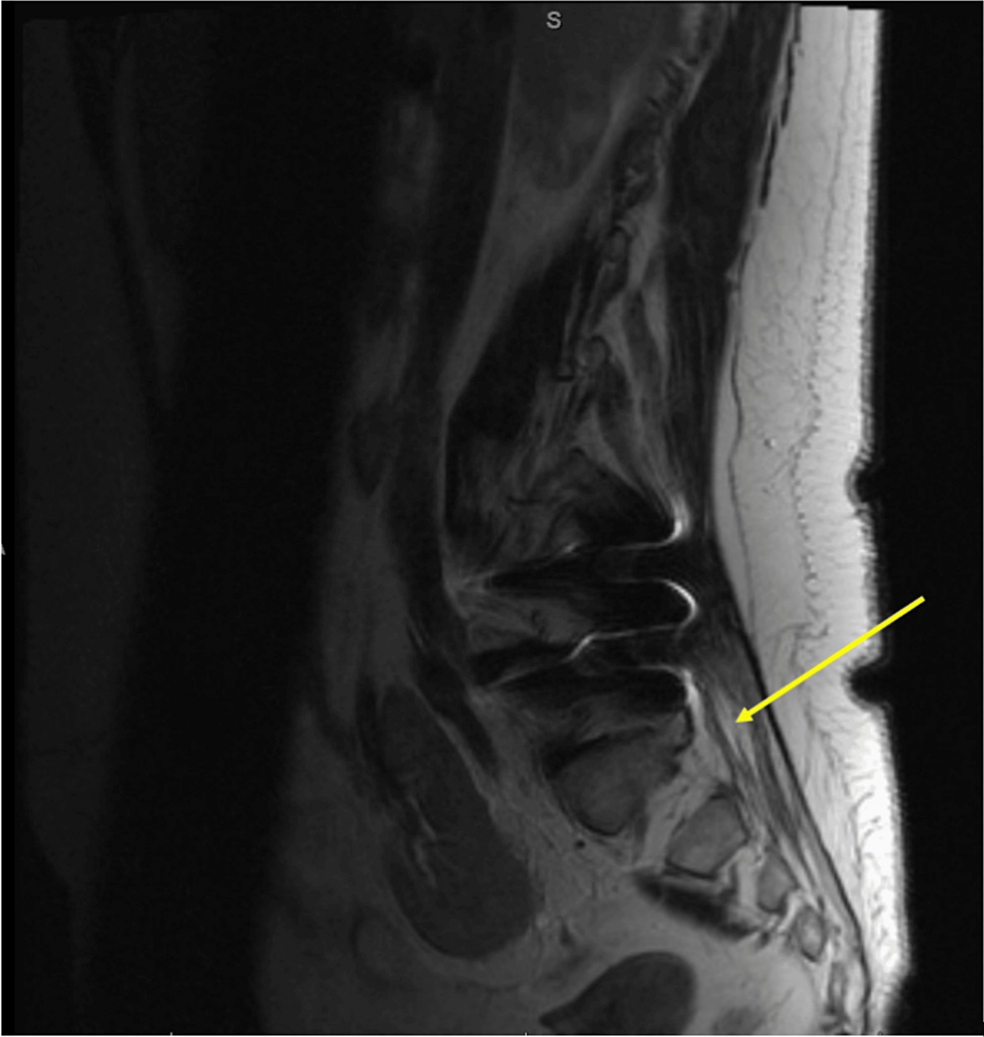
Lumbar MRI with and without gadolinium demonstrates postsurgical and degenerative changes as well as diffuse enhancement of all visualized cauda equina nerve roots. MRI: magnetic resonance imaging.
